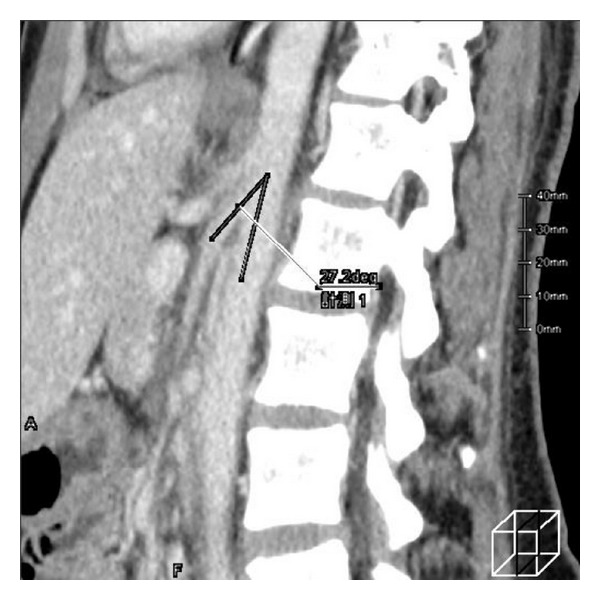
Enhanced computed tomography of sagittal section. The angle between the aorta and the SMA was 27.2 degrees.
